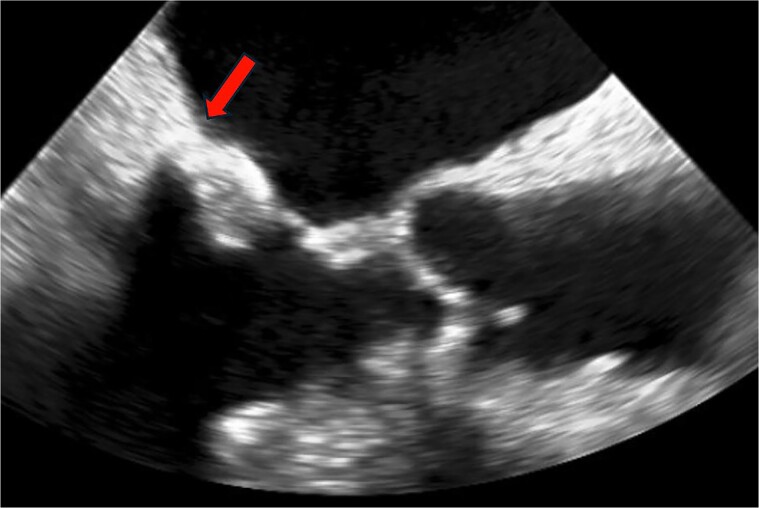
Transoesophageal echocardiography imaging of caseous calcification of the mitral annulus. The arrow indicates the mass-like appearance of caseous calcification of the mitral annulus.
